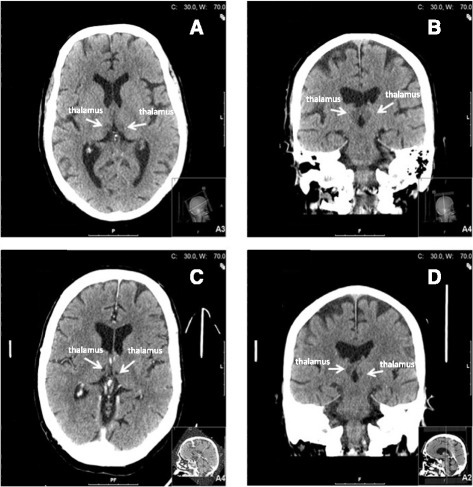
a Head computed tomography (axial) performed on the day of admission was initially assessed as normal, although signs of bilateral ischemia in the thalami actually were visible. b Head computed tomography (coronal) performed on the day of admission was initially assessed as normal, but later re-evaluated to be bilateral ischemia in the thalami. c Head computed tomography (axial) performed on day 24 of hospitalization showed bilateral ischemia in the medial areas of the thalami. d Head computed tomography (coronal) performed on day 24 of hospitalization showed bilateral ischemia in the medial areas of the thalami
